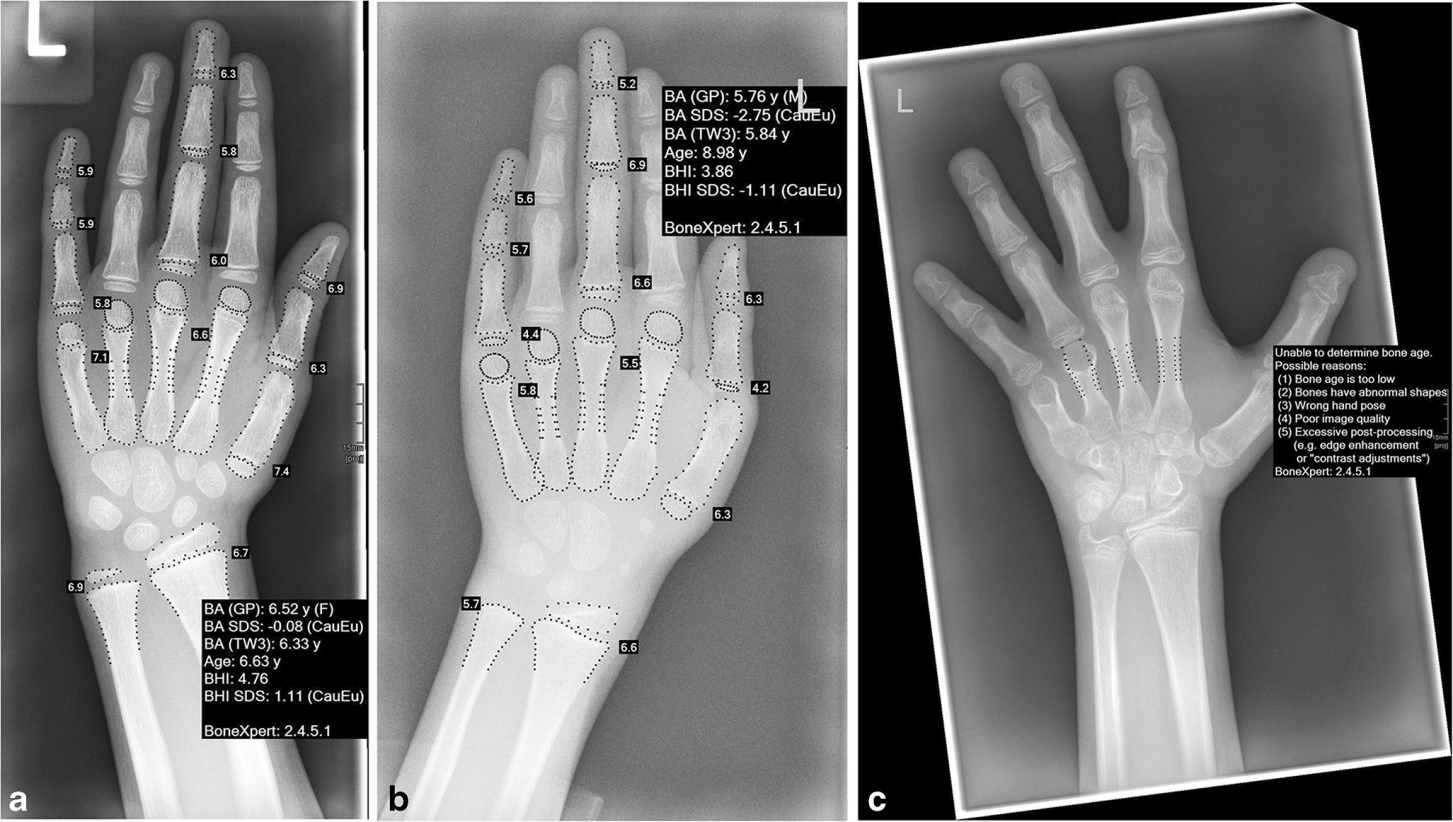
BoneXpert interpretation of left-hand radiographs. a Dorsopalmar left hand and wrist in a 6-year, 7-month-old girl with hypophosphatasia. Her bone age is within normal limits (0.08 standard deviations below the mean). b Dorsopalmar left hand and wrist in an 8-year, 11-month-old boy with short stature. His bone age is delayed (2.75 standard deviations below the mean). c Dorsopalmar left hand and wrist in an 11-year, 8-month-old girl whose radiograph shows short fourth and fifth metacarpals, previous cone-shaped epiphysis of the middle phalanx of the index finger and short terminal phalanges. BoneXpert was not able to determine bone age in this child with dysmorphic bones
